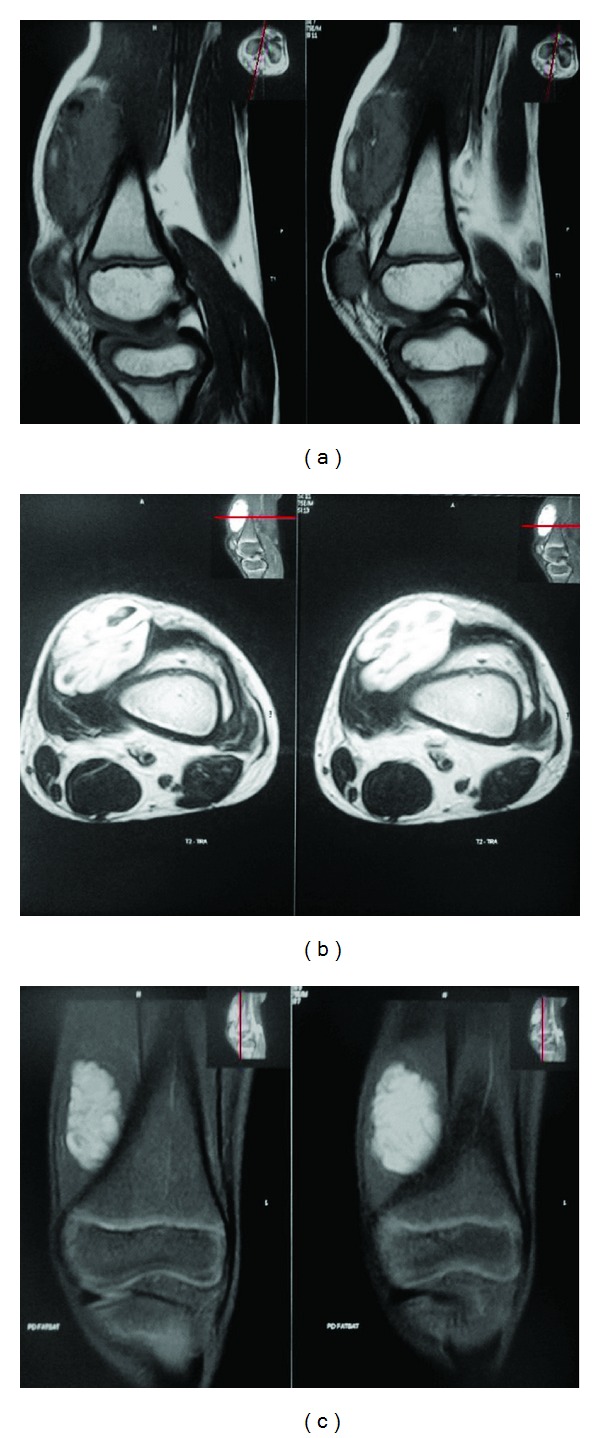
MRI reveals soft tissue mass in the vastus medialis which with high signal intensity on *T*
